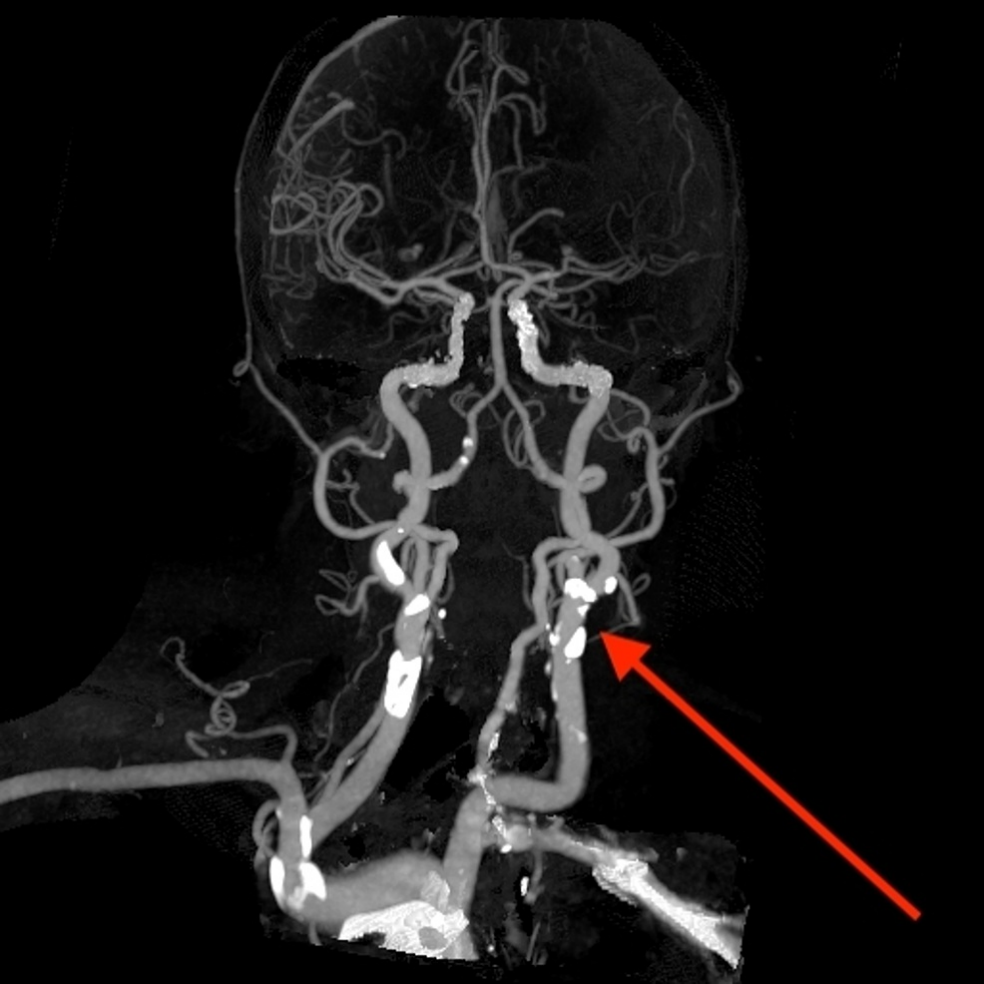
CT angiogram of the head and neck shows showing irregular calcified atherosclerotic plaques.
